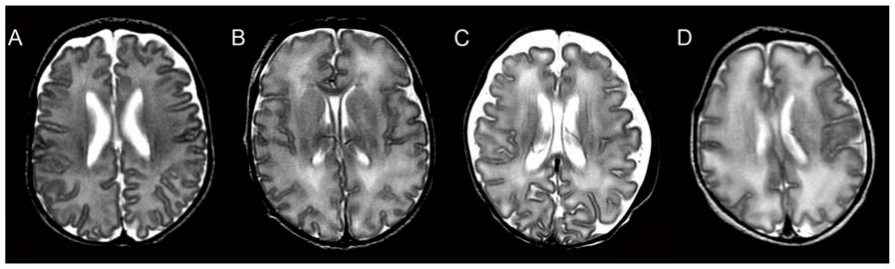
T2-weighted images for each neonate were rated for DEHSI. Examples of cases rated as “within normal limits (WNL)” (A), mild DEHSI (B), moderate DEHSI (C) and severe DEHSI (D) are depicted above. All 108 cases were rated independently for DEHSI by two investigators who used a modified version of the scale by Maalouf and colleagues (Table S1) [11].
